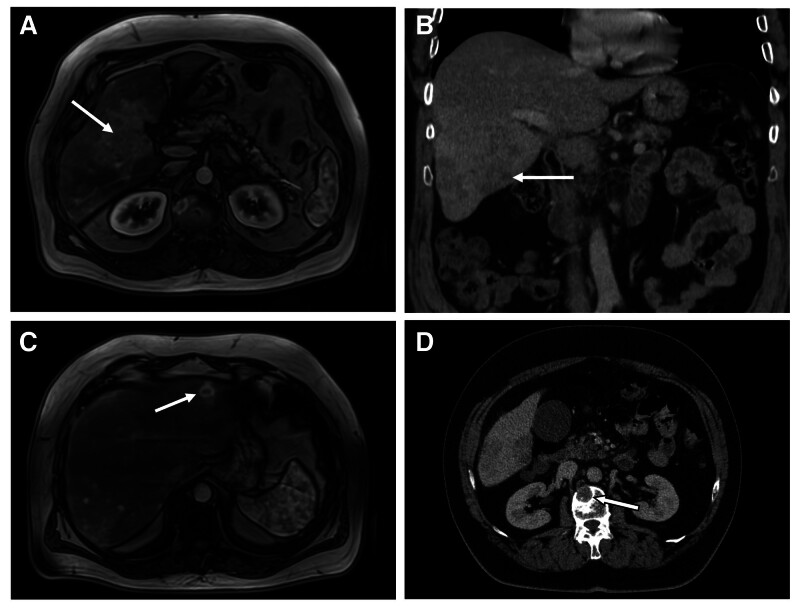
(A) Abdominal magnetic resonance imaging (MRI) showed an intrahepatic mass in the right lobe (segment IV/V). (B) Computed tomography (CT) scan of the same intrahepatic mass subsequently diagnosed as primary hepatic cholangiocarcinoma. (C) MRI revealed metastatic lesions in the left liver lobe (segment II/III). (D) CT scan showed an osteolytic bone metastasis in the lumbar spine (L2).
